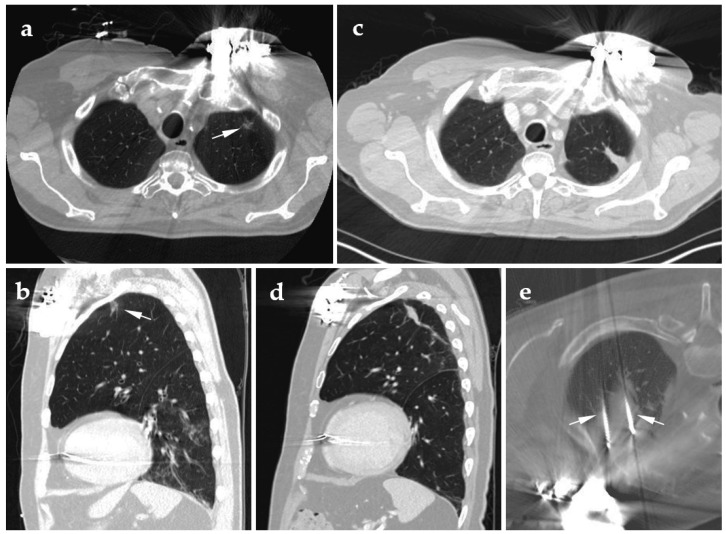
78-year-old male with no significant comorbidities had biopsy-proven lepidic adenocarcinoma consistent with stage 1 NSCLC. As his automatic implantable cardioverter defibrillator would have needed to be removed for SBRT treatment, he was referred to interventional radiology. (a) Supine axial pre-ablation CT with the white arrow indicating the lesion to be ablated; (b) supine sagittal pre-ablation CT with the white arrow indicating the lesion to be ablated; (c) supine axial follow-up CT at 3 years post-ablation; (d) supine sagittal follow-up CT at 3 years post-ablation; (e) prone axial CT shows the two probes (white arrows) bracketing the lesion during the tumor cryoablation.
